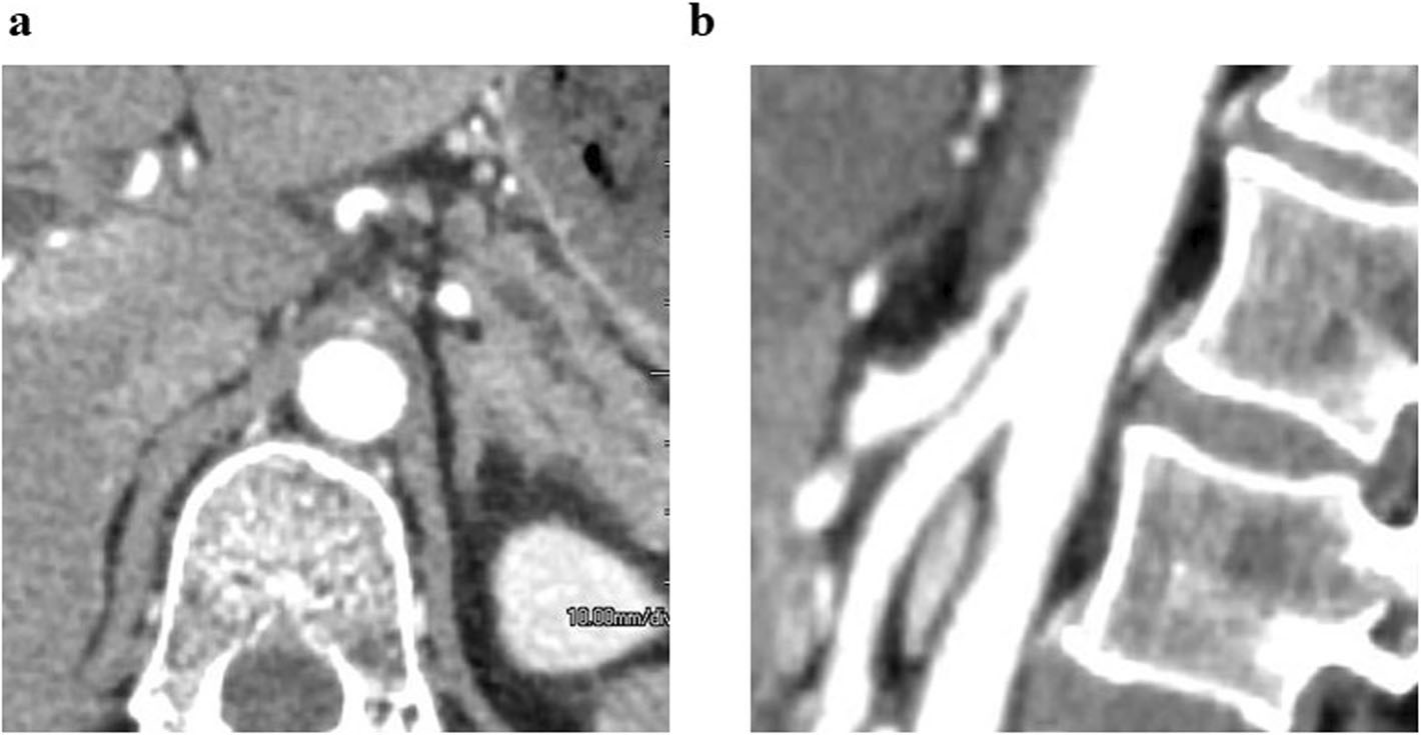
CACS due to MALS (case 3). Preoperative enhanced CT
